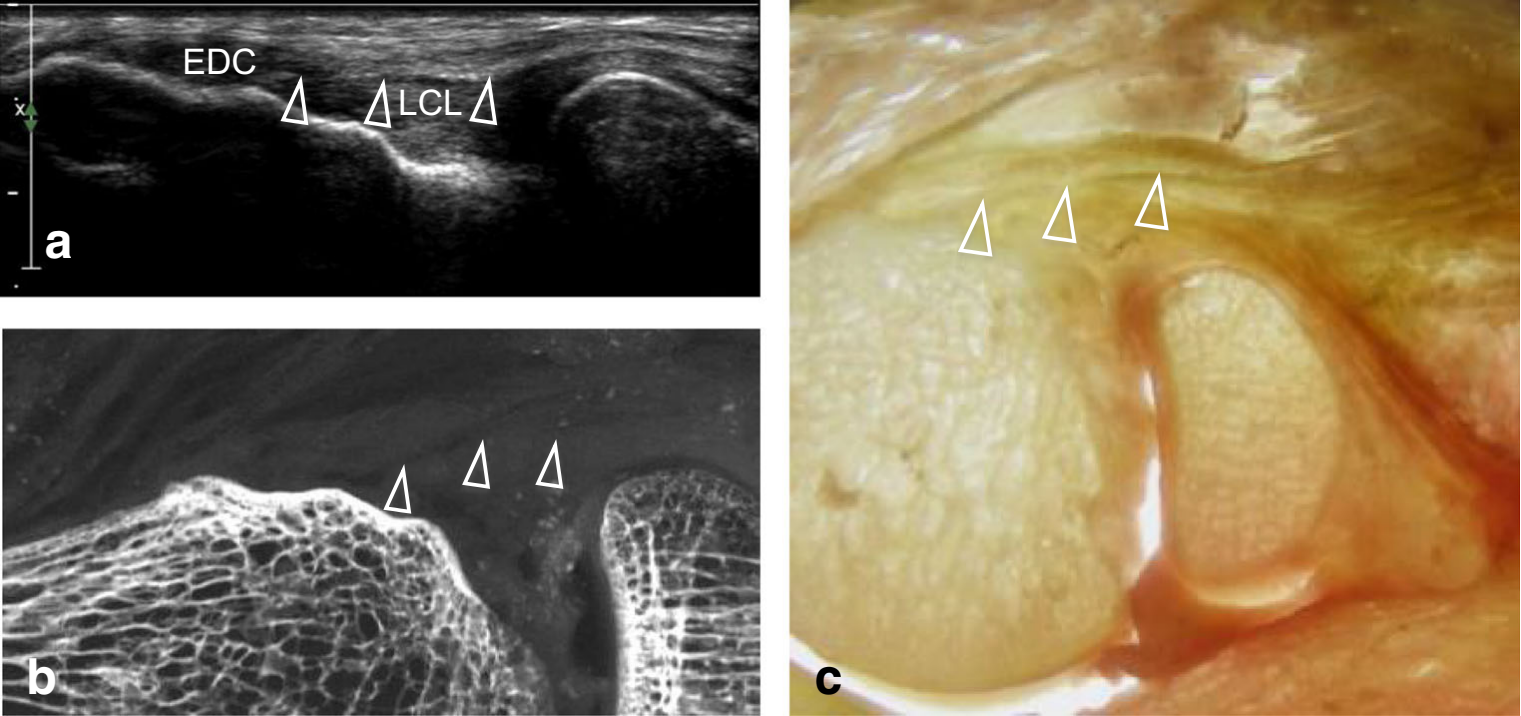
Identification of a hyperechogenic line between the lateral collateral ligamentous complex (LCL) and the overlying extensor digitorum communis (EDC) tendon at ultrasonography (a) corresponding to a radiolucent line on radiograph (b), likely corresponding to a fat layer (arrowheads). The interface is also seen on the backlit gross anatomical slice (c)
